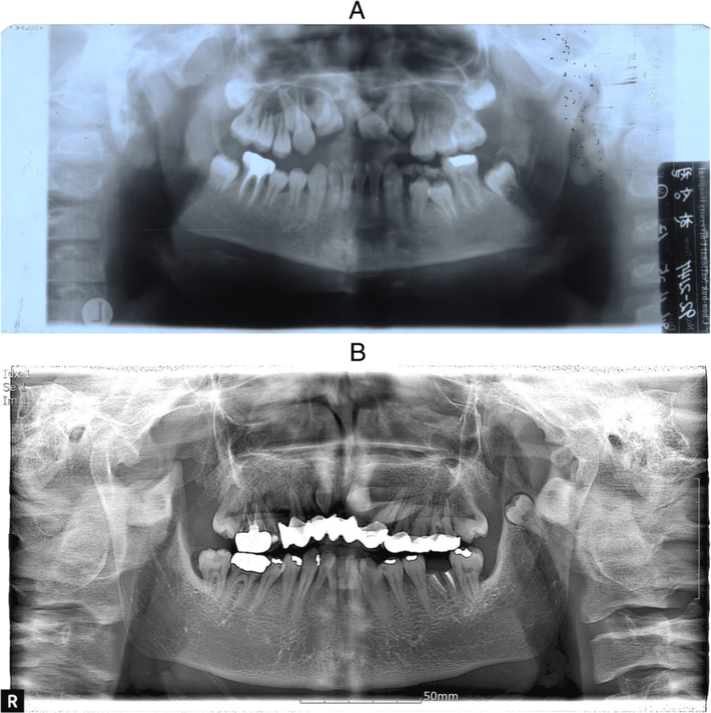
Panoramic radiographs at the age of 11 and 29 show unerupted maxillary permanent teeth. a At the age of 11. b At the age of 29
